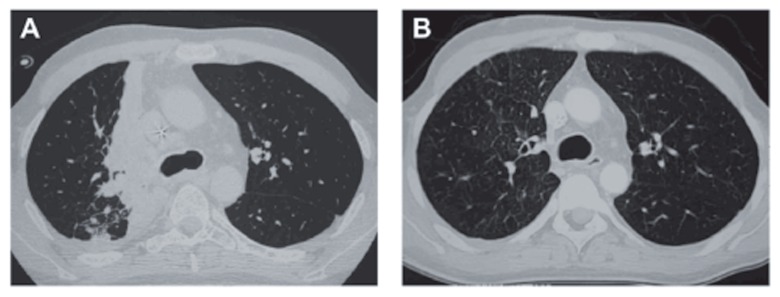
Evolution of lung computed tomography scan from patient 1. A) Eighty four days after cord blood transplantation, the scan showed a right hilar mass together with an atelectasis of the right upper lobe leading to the diagnosis of endobronchial post transplant lymphoproliferative disorder. B) Five months later, while the patient had received 3 courses of rituximab and cytotoxic chemotherapy, the scan was normalized.
